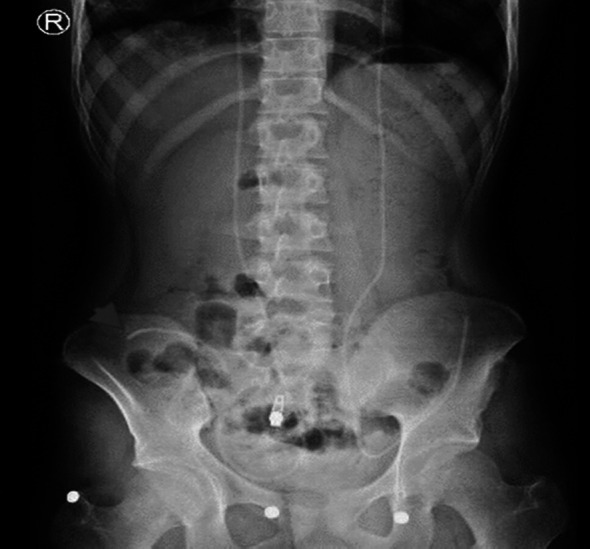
Plain AP abdomen radiograph demonstrates bilateral VPS peritoneal catheters with the tip of the right catheter in the right iliac fossa (arrowhead).
